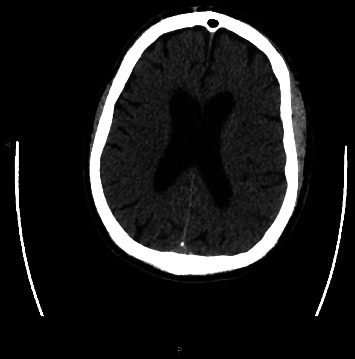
Postcontrast CT with iodine subtraction, noting the absence of contrast enhancement compared to Figure 1.
